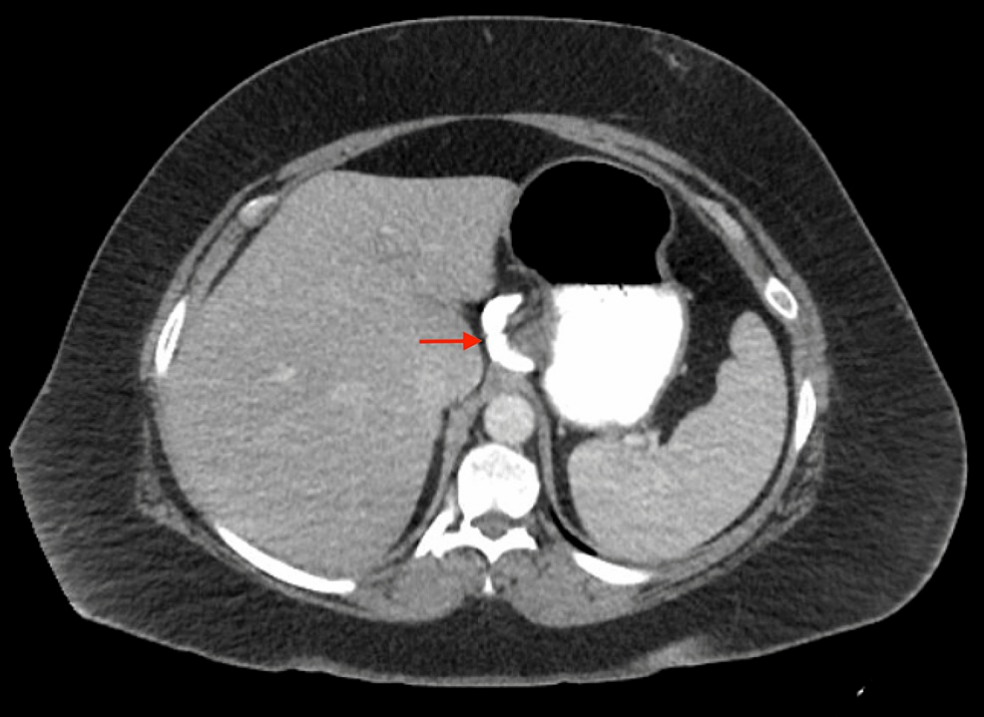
CT abdomen and pelvis with oral and intravenous contrast, with the red arrow pointing to an appropriately placed gastric band.
